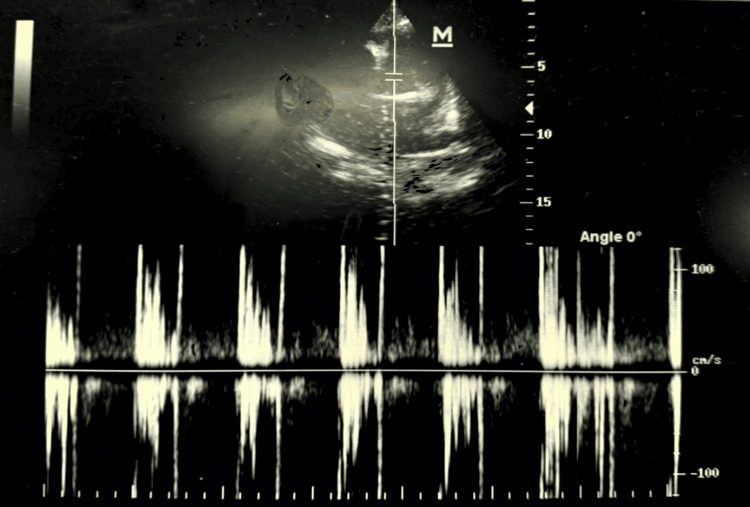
ECHO finding the patient showing a mild mitral regurgitation ECHO: echocardiogram
